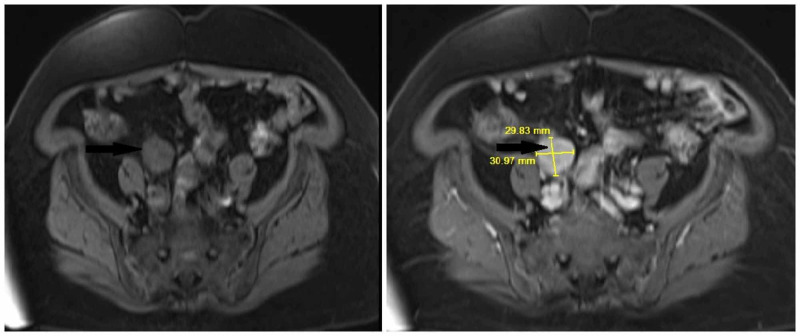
MRI of abdomen W/O and W/contrast showing similar appearing lesion in right pelvis just anterior to the common iliac arteries measuring 3.1 x 3.0 cm (arrow).
